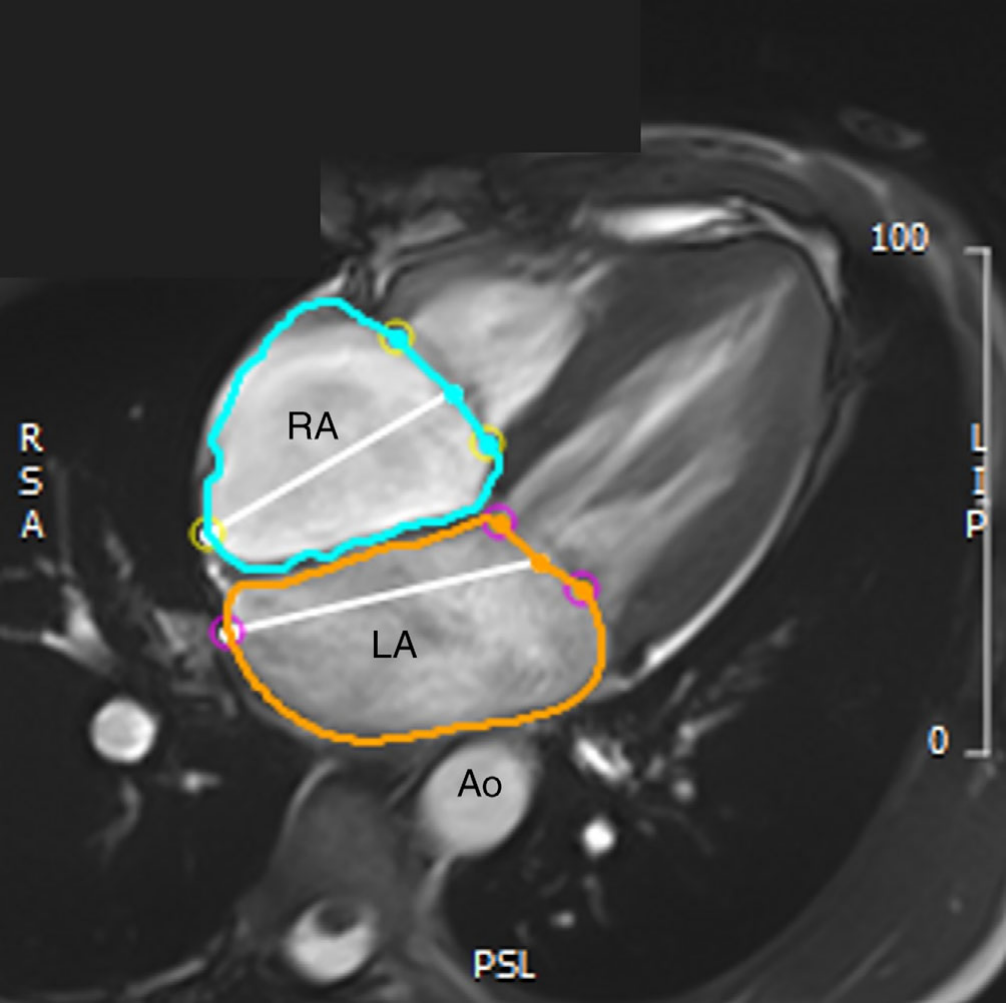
Example of the manual delineation of the left (orange) and right (light blue) atrium with cardiac magnetic resonance imaging (cMRI). The LA includes the left atrial appendage, but excludes the pulmonary vein, while the RA includes the right atrial appendage, while excludes the superior and inferior vena cava. Ao, aorta; LA, left atrium; RA, right atrium
